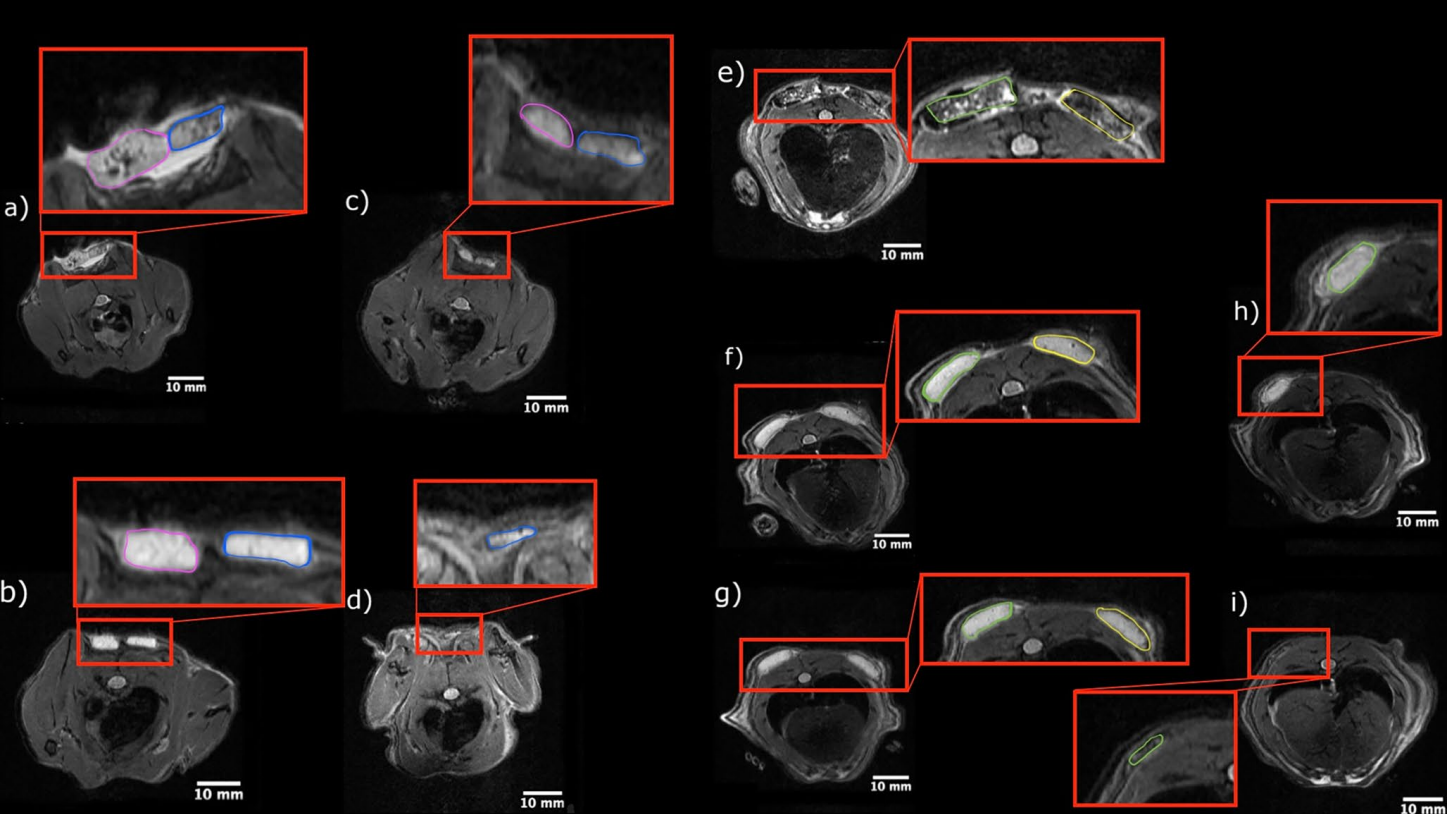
Representative MRI images (T2 sequence) of implanted collagen membranes. The visualized regions were zoomed in for clarity, and membranes are delineated with colored outlines corresponding to each membrane type: membrane A (violet), membrane B (blue), membrane C (green), and membrane D (yellow). Images are shown for Day 1 (a, e), Day 7 (b, f), Day 14 (c, g), 1 month (d, h), and 2 months (i). Membrane A is no longer visible at day 28 (d), and membrane D is undetectable by 2 months (i).
